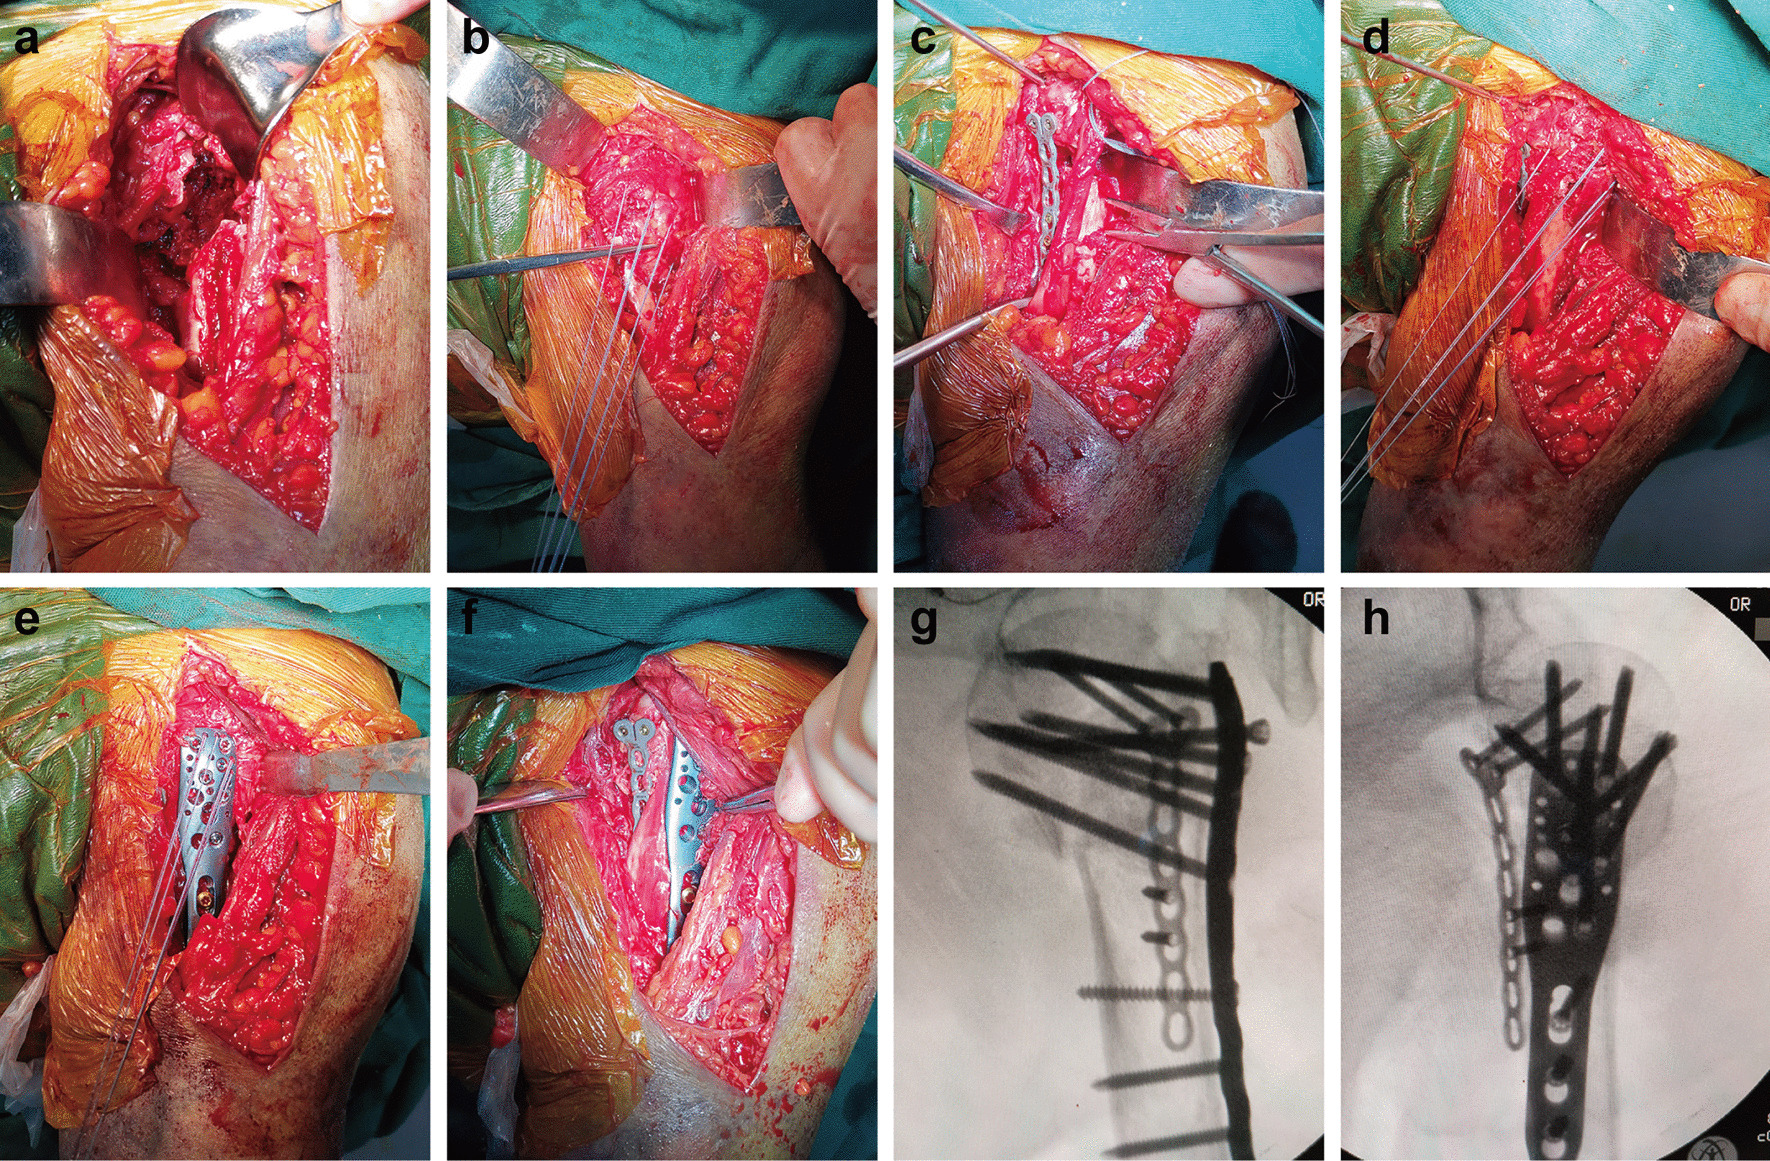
Surgical procedure involved in the reduction technique in one humeral proximal fracture with medial instability. a Images obtained during operation revealed comminution of the lateral cortex. b The suture technique was used to restore the alignment of the fractures. c A T-shaped 2.7-mm locking plate was placed at the medial side of LHBT, with the T-shaped head fixed on the lesser tuberosity and the distal end fixed on the shaft. d By using the anteromedial locking plate, the lateral cortex was easily reconstructed with the suture technique and a joystick. e, f PHILOS was placed lateral to LHBT, and the traction sutures were passed through the suture holes in order to reduce the tuberosities prior to locking screw insertion and finally tied. g, h The AP and lateral radiographs of the shoulder joint were captured and attention was paid to the use of calcar screws to further strengthen the medial support
